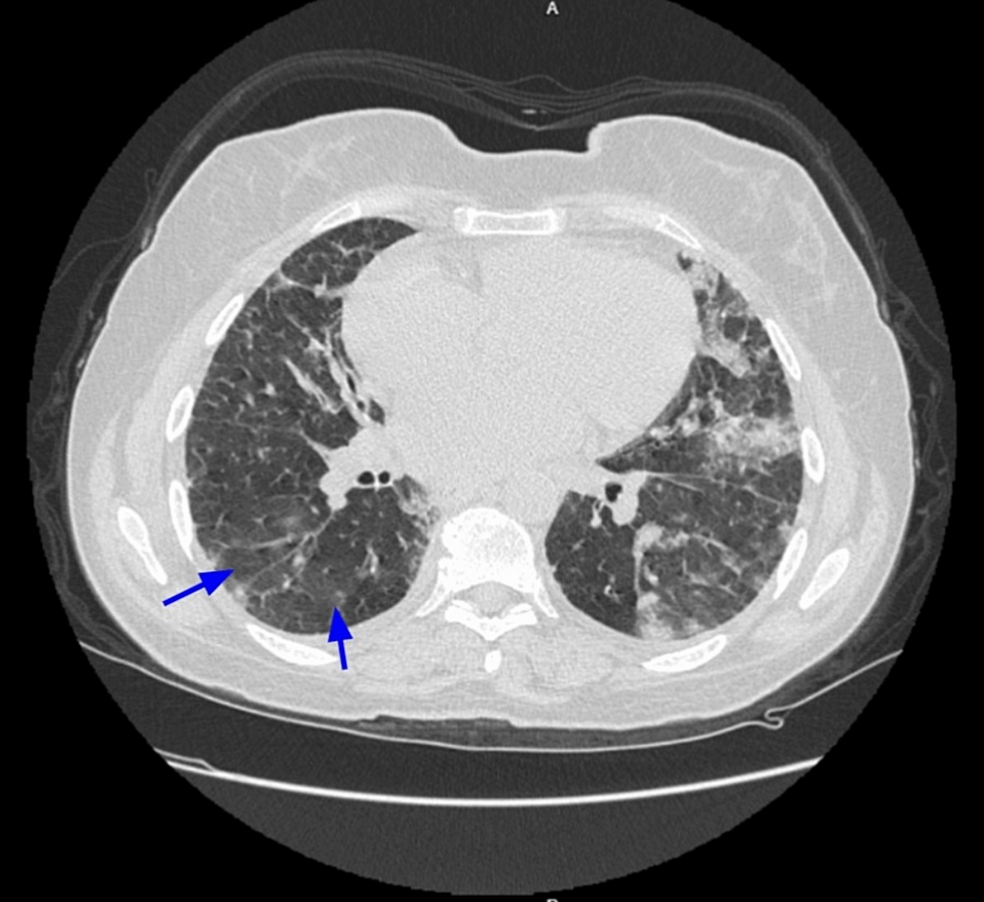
HRCT of the chest HRCT of the chest showing ground-glass opacities in the lung parenchyma (blue arrows).
